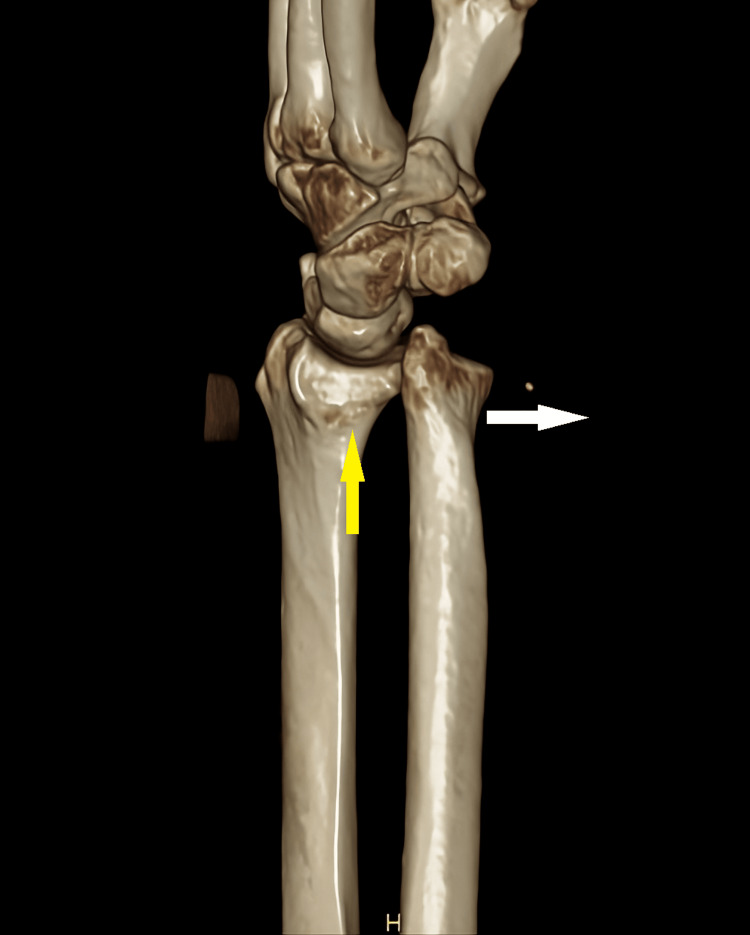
CT scan with 3D reconstruction of the right wrist showing the ulna displaced volarly (direction of white arrow) and an empty sigmoid notch of the radius (yellow arrow). CT: computed tomography, 3D: three-dimensional
